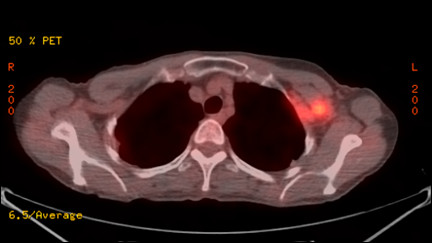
FDG-PET showed swelling of the left axilla and infraclavicular lymph nodes with FDG accumulation (SUV max 4.5). No accumulation was observed in other organs, including the left breast.
